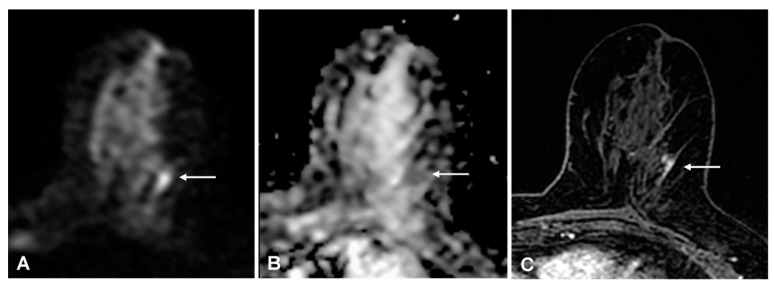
(A) 54-year-old woman with a 15-mm invasive ductal carcinoma: true-positive finding on diffusion-weighted imaging (DWI) that was detected by expert readers only. A, DWI (b = 1000 s/mm^2^) shows a mild linear hyperintense lesion in the upper outer portion of the left breast (arrow). (B) apparent diffusion coefficient-map shows corresponding homogeneous hypo-intensity. (C) for comparison, the axial T1-weighted fat-sat contrast-enhanced image shows focal non-mass enhancement in the same location (arrow).
